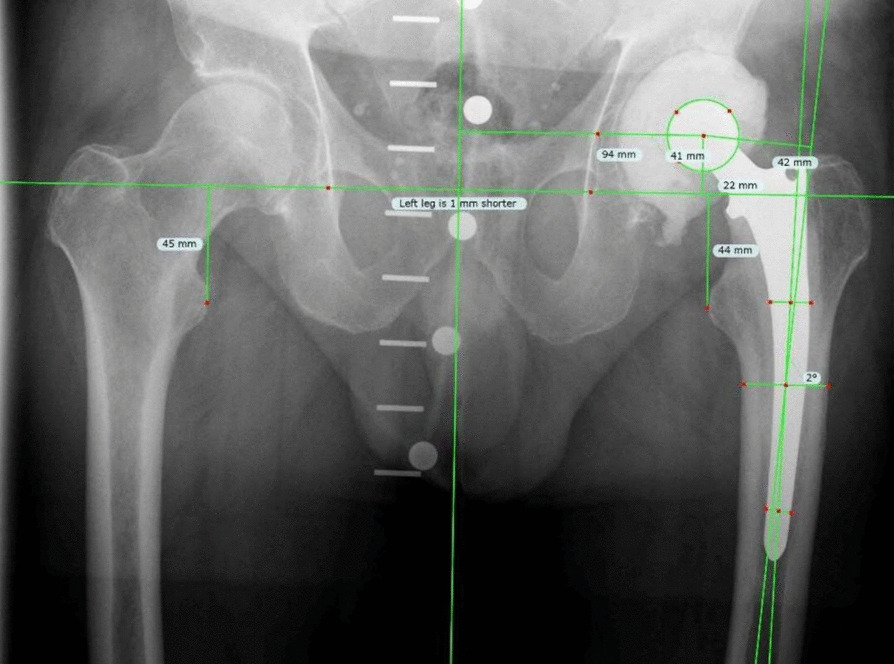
Anteroposterior pelvic radiograph demonstrating a precise restoration of patient-specific hip biomechanics after implantation of the custom-made articulating hip spacer. The custom-made spacer allows accurate reconstruction of femoral offset and center of rotation, resulting in near-symmetric limb length and physiological alignment. This is essential to maintain soft-tissue tension, optimize function, reduce instability, and facilitate subsequent second-stage reimplantation
